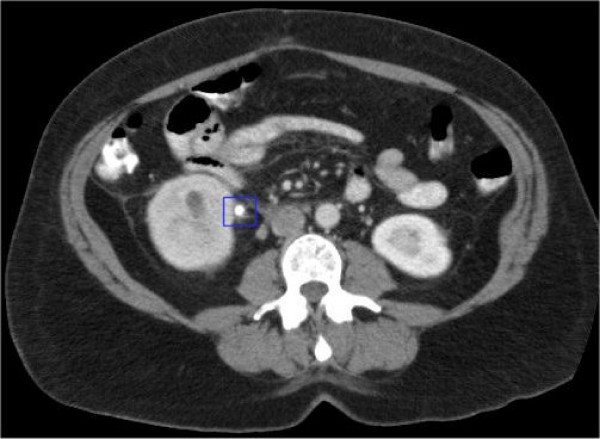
CT scan showing an 8 mm calculi at the right PUJ.
